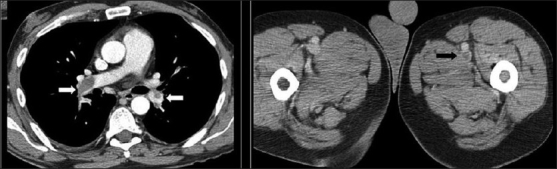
Thromboembolism. Computed tomography (CT) images from a 38-year old human immunodeficiency virus-positive man with a CD count of 400 presented with acute tightness in the chest and low O_2_ saturation. The CT scans show pulmonary emboli within the lower lobe pulmonary arteries on both sides (white arrows). Note the thrombus in the left femoral artery (black arrow)
